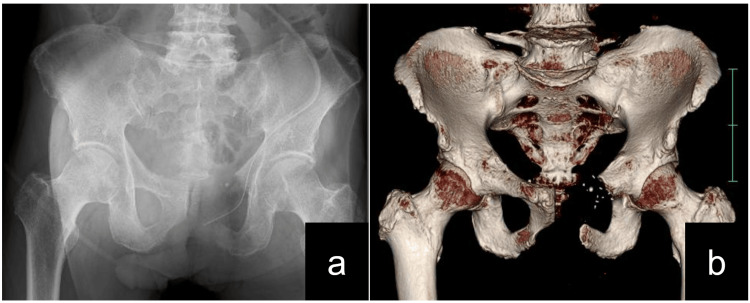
After a type 3 internal hemipelvectomy. (a) Postsurgical radiograph following a type 3 internal hemipelvectomy. (b) Three-dimensional computed tomography postoperative image after a type 3 internal hemipelvectomy.
